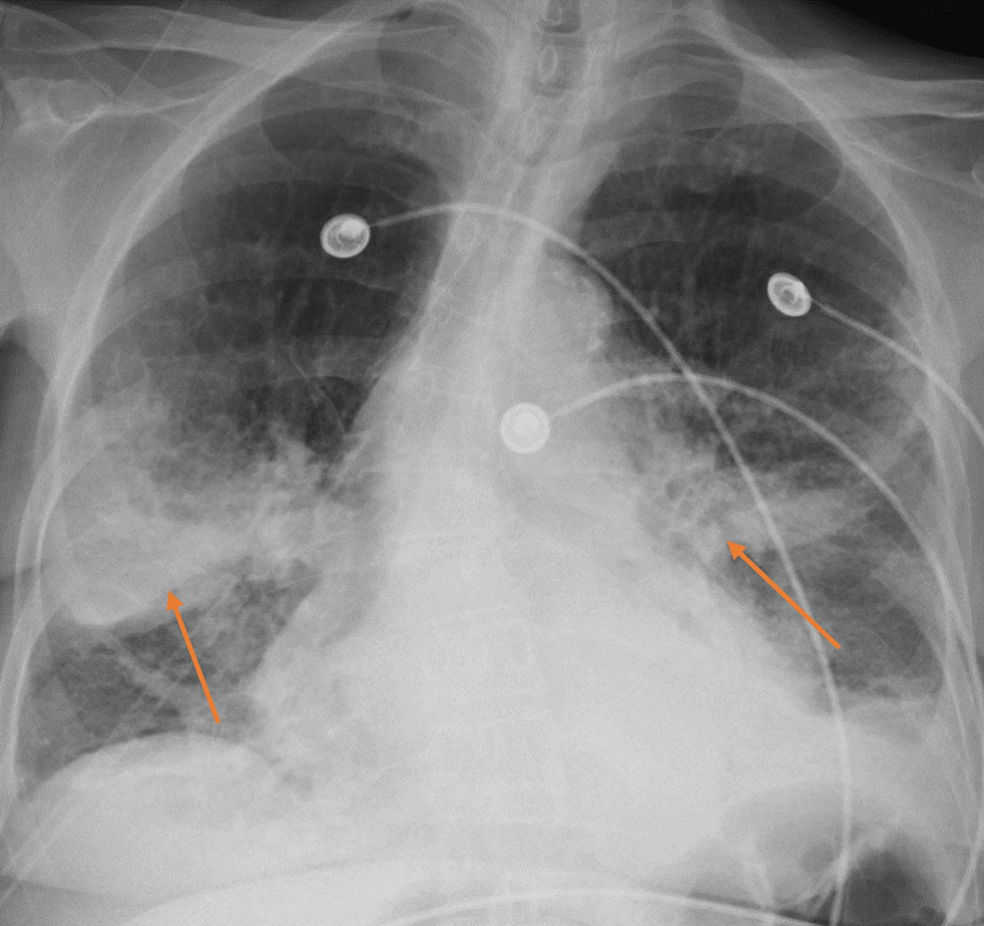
Chest X-Ray Demonstrating Right Middle Lobe and Superior Left Lower Lobe Infiltrates (arrows).
